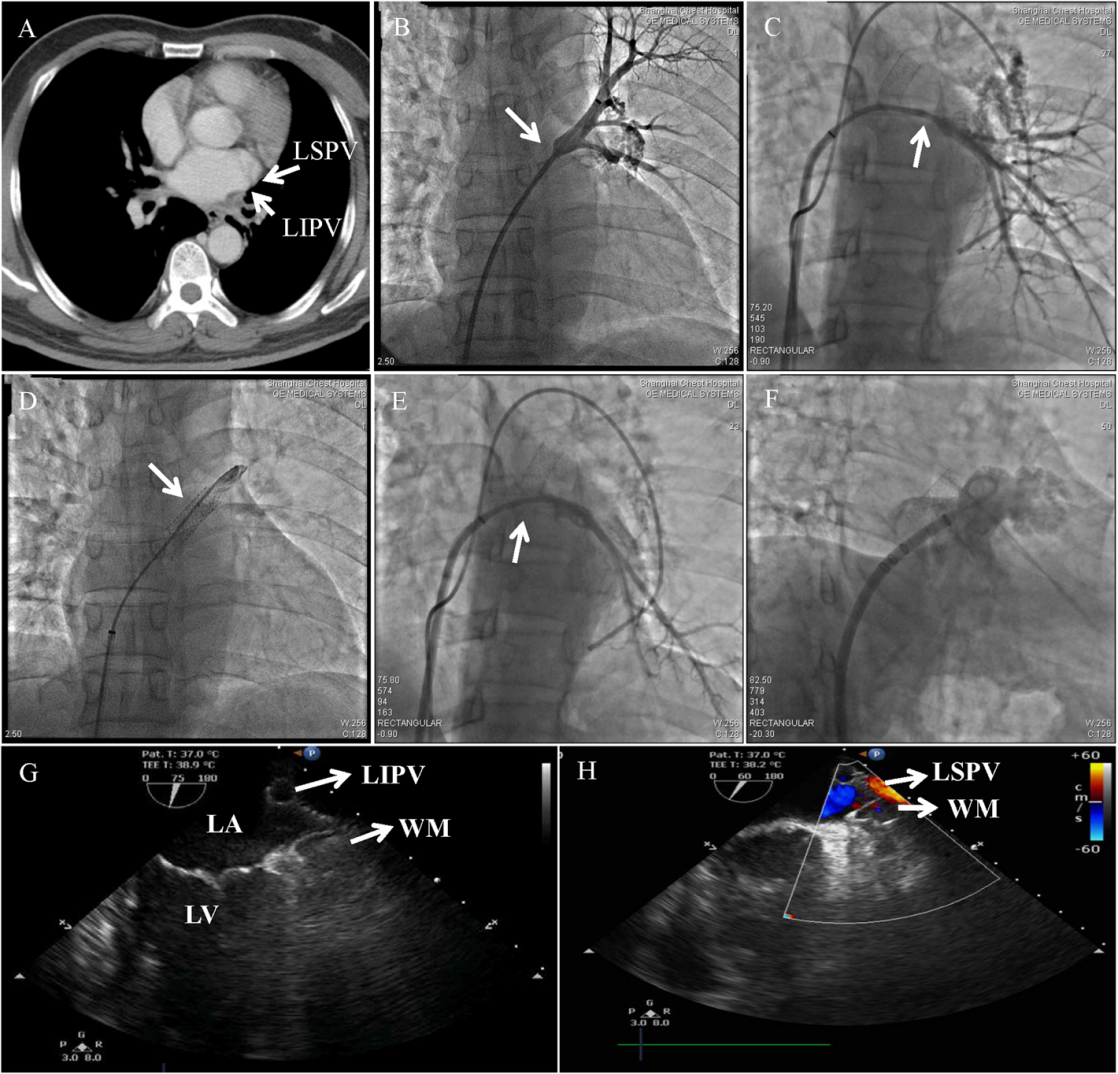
a Subtotal stenosis of the left PV in CT scan. b, c PV angiography shows severe stenosis of the left PV (arrow) and plexiform vein collaterals before PV Stenting. d, e PV angiography shows patent stents with no residual stenosis (arrow) after PV Stenting. f Left atrial appendage angiography. g, h Partially deployed Watchman device
